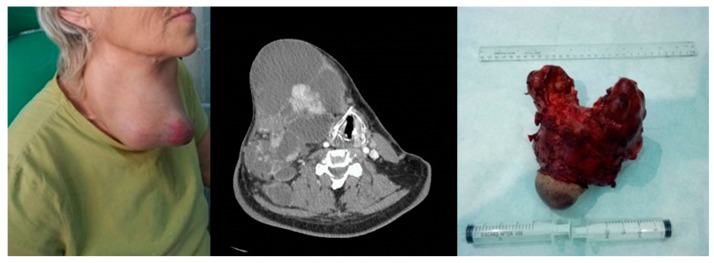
Anaplastic thyroid carcinoma. Left image—clinical aspect. Center image—axial CT scan with tumor displacement of the airway. Right image—resection piece 750 g.
